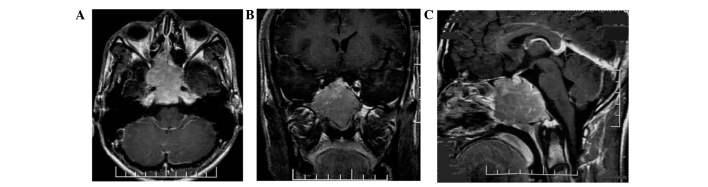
(A) Axial, (B) coronal and (C) sagittal section of T1-weighted magnetic resonance imaging with contrast revealing an expansive tumor originating from the clivus and surrounding both cavernous sinuses, compressing the front of optic chiasm in the sphenoid sinus area of the middle fossa.
